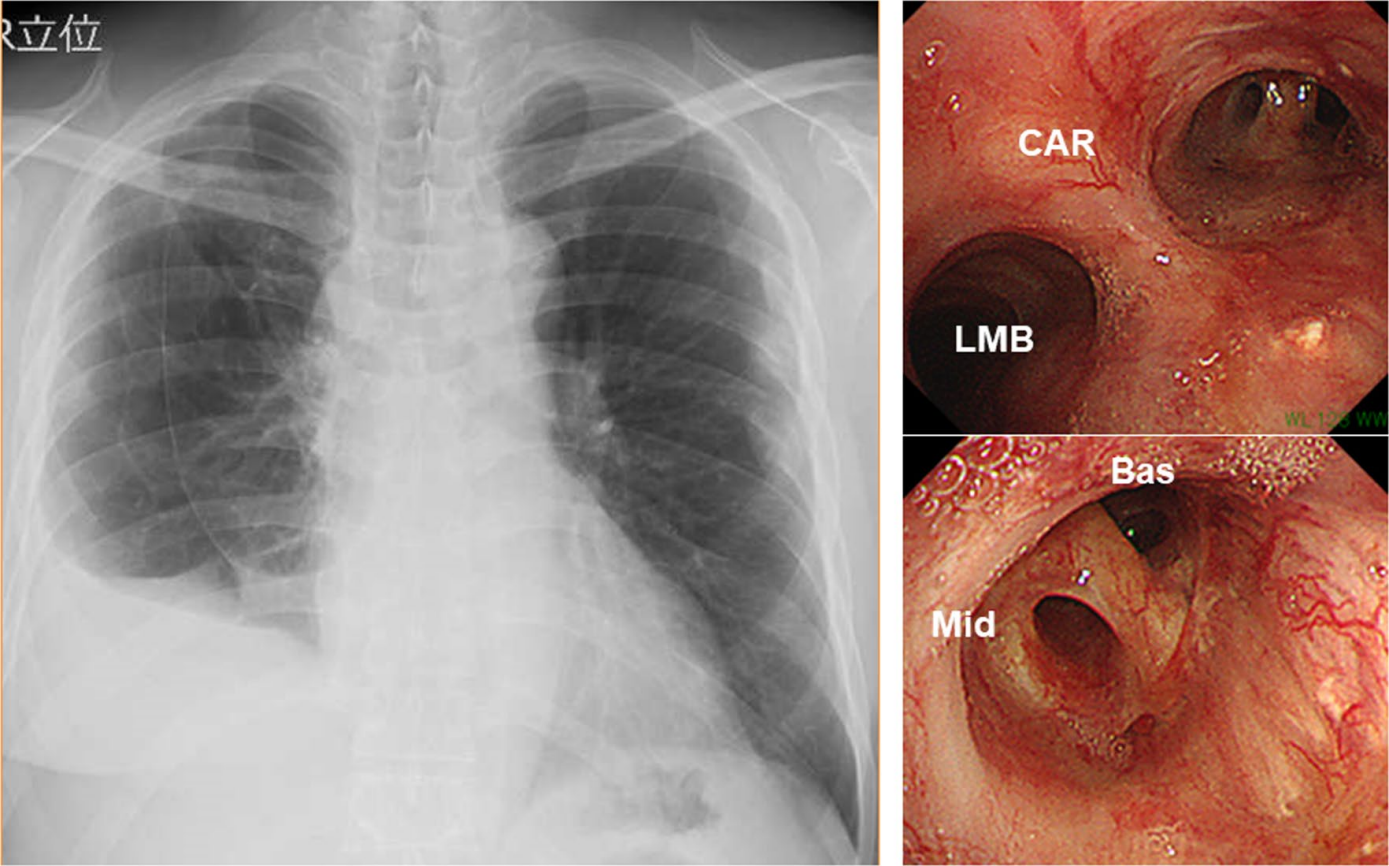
Chest roentgenogram (left) and bronchoscopy (right) at 4 months after surgery showed excellent lung expansion and bronchial healing. *CAR* carina, *LMB* left main bronchus, *Mid* right middle bronchus, *Bas* right basal bronchus
